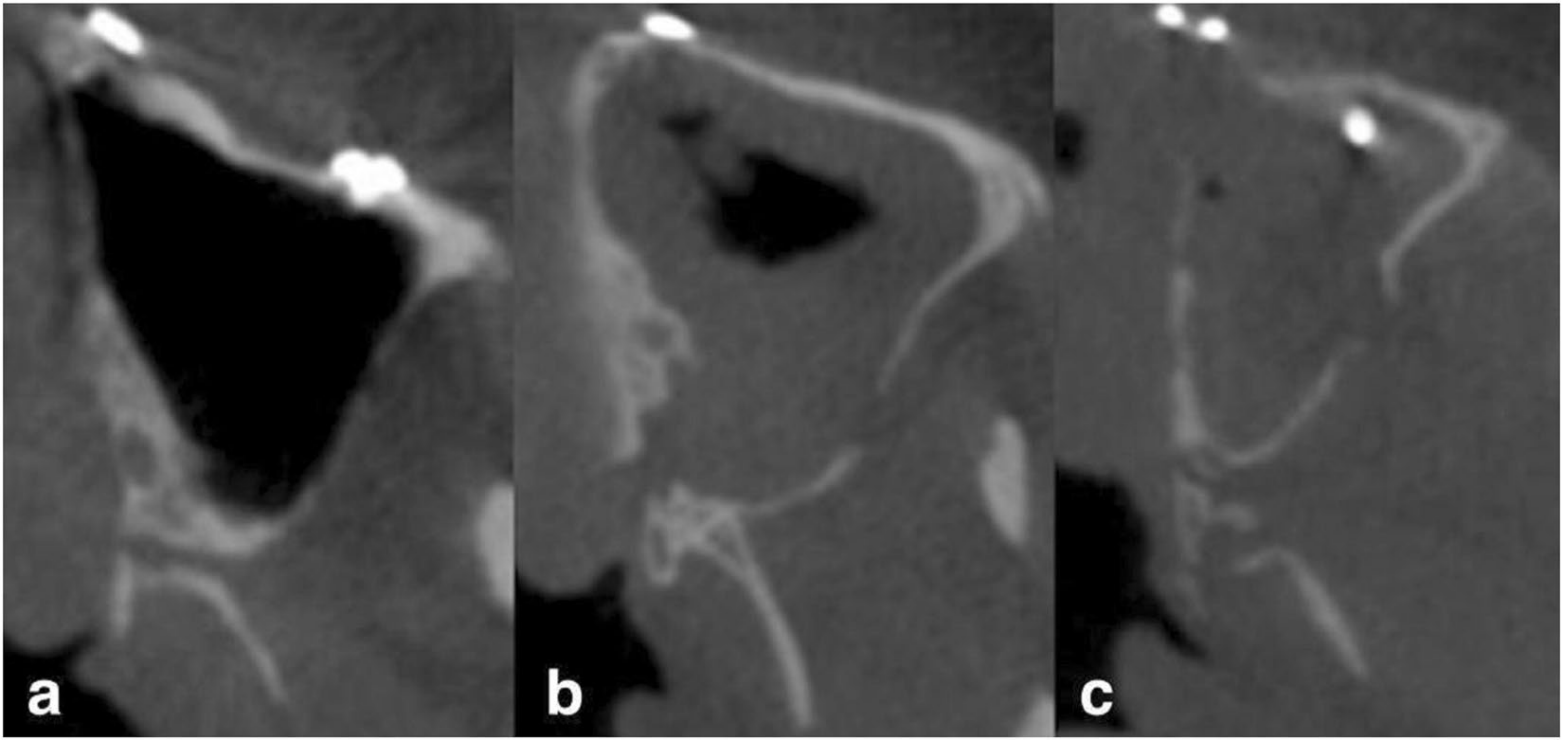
Pterygomaxillary separation type in postoperative CBCT. (a) Clean cut type: the cutting line within the PMJ. (b) Maxillary sinus type: part of the posterior wall of maxillary sinus attached to the PMJ after separation. (c) Pterygoid plate fracture type: pterygoid fracture occurred after PMJ separation.
